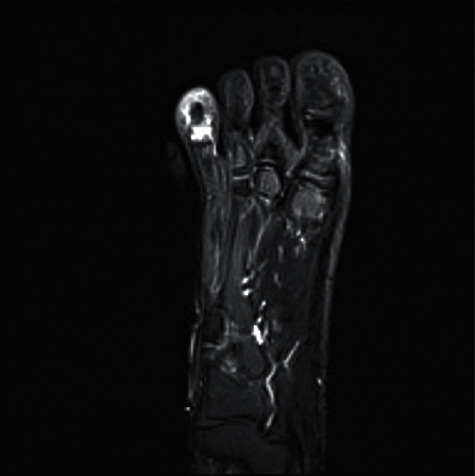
T2-weighted image on MRI, showing the sclerotic nidus hyposignal and the surrounding inflammation of the soft tissues around (hypersignal).
